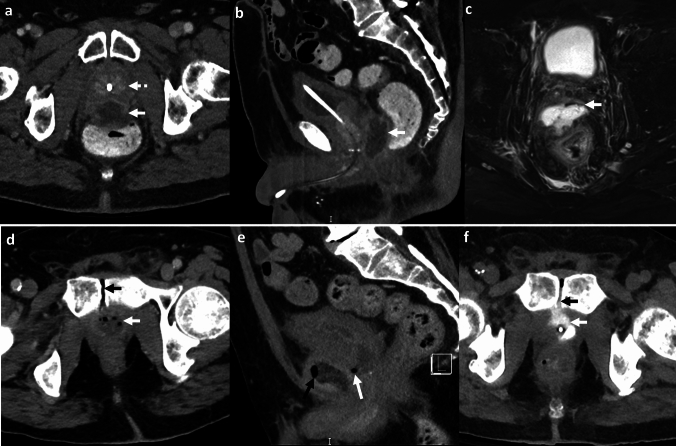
Pitfalls for emphysematous prostate abscess. a–c Infected SpaceOAR: A 65-year-old with prostate cancer and recent SpaceOAR hydrogel insertion presented with fever and difficulty urinating, CT images a, b show a rim-enhancing lesion in the recto-prostatic region (solid white arrow, a, b) separate from the prostate (dotted white arrow, a). MRI shows a small air-fluid level anteriorly (solid white arrow, c) this was a proven infected SpaceOAR. Erosion into the rectum can also lead to air within the SpaceOAR. d–f Urosymphyseal fistula: A 71-year-old with prostate cancer and prior radiation therapy with sepsis. CT image d shows prostate abscess with air (solid white arrow, d, e) and air in the pubic symphysis (black arrow, d, e). Subsequent excretory phase CT image f shows excreted urinary contrast filling the prostate cavity (solid white arrow, f) and communicating with the pubic symphysis (black arrow, f) consistent with urosymphyseal fistula
